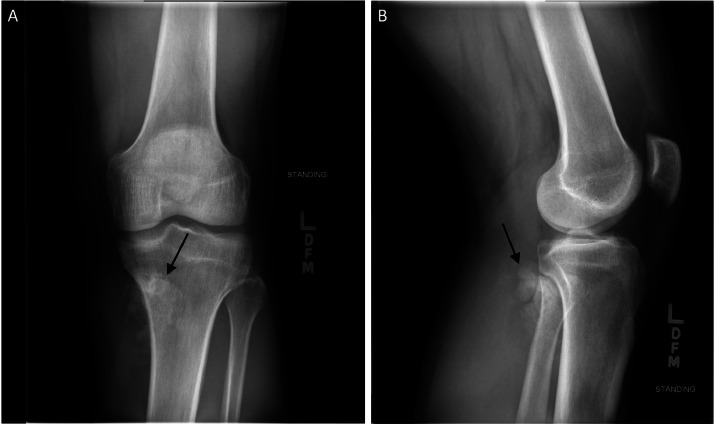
Radiographs of the left knee. (A) Anteroposterior radiograph shows poorly defined osseous mineralization (arrow) about the proximal medial tibia. (B) Lateral radiograph shows an exophytic ossified mass arising from the proximal posterior tibial cortex.
